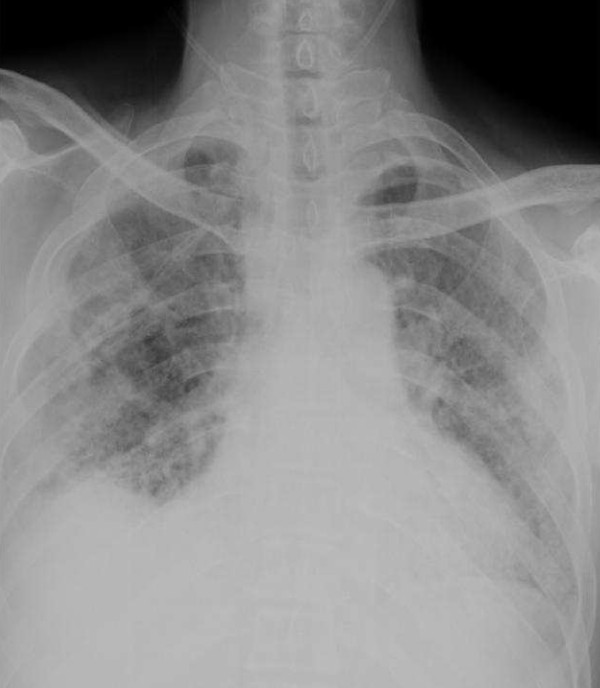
Chest radiograph showing extensive interstitial infiltration compared to previous chest radiograph.
